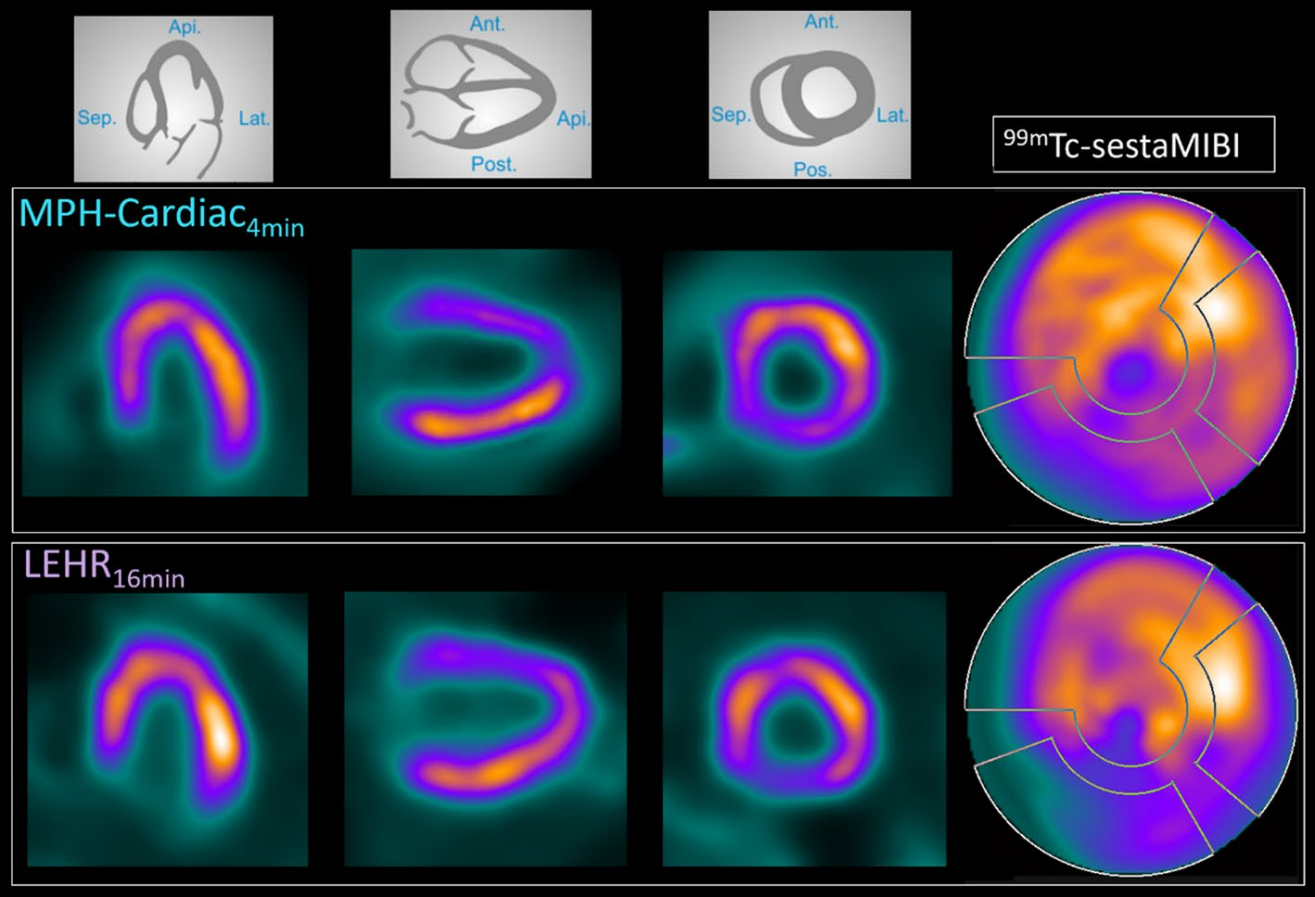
Representative post-stress SPECT image slices and polar maps of a 67 years old male CAD patient. Reconstructed images of the four minutes MPH-Cardiac acquisition (upper row) and sixteen minutes LEHR acquisition (lower row)
